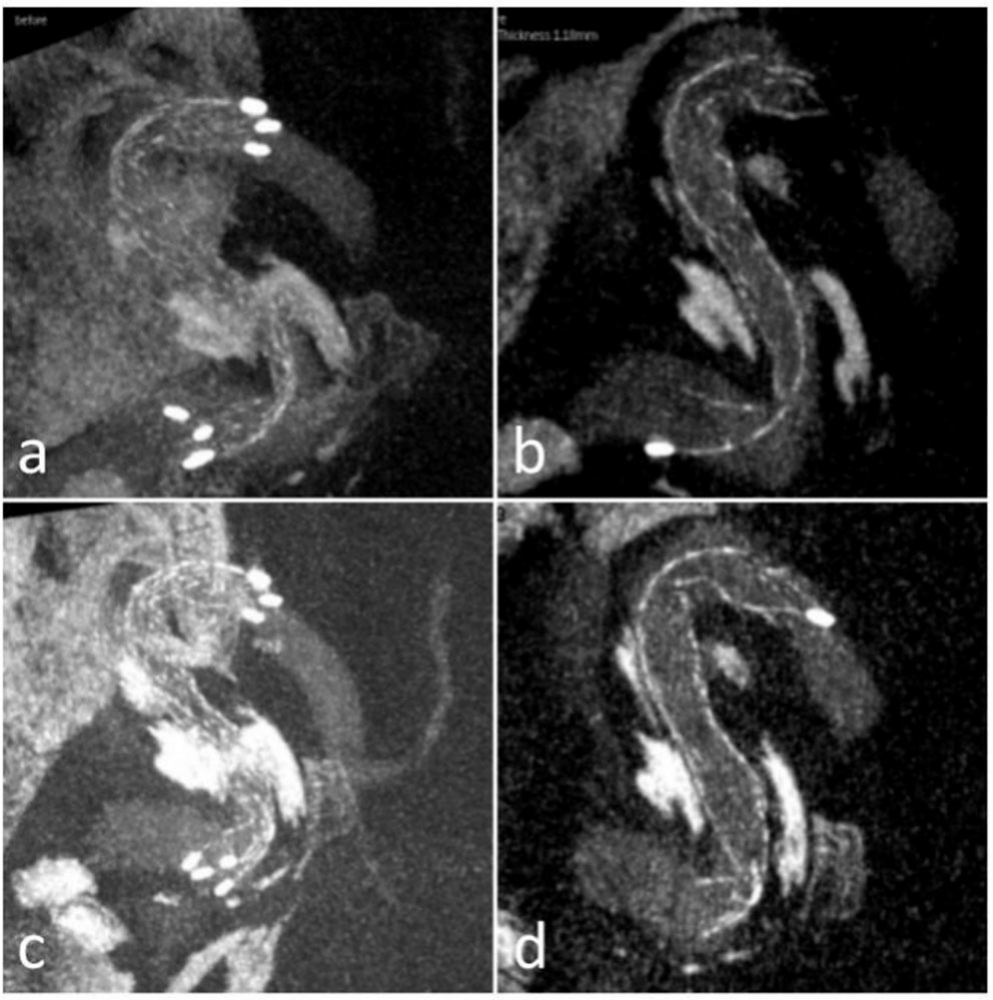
Case 23 (a,b) sagittal views of the stent in Xper CT during the procedure showed the kinking; (c,d) sagittal views of the stent after massaging via microcatheter or microwire showed the kinking improved.
